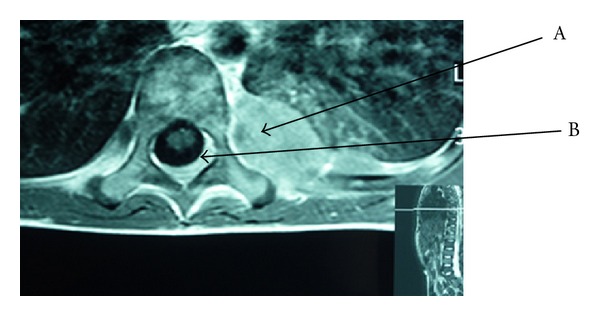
MRI (axial T1): dorsal paravertebral mass (T7) (A) with extra dural deposits and compressin of the thecal sac (B) from tumor spread.
